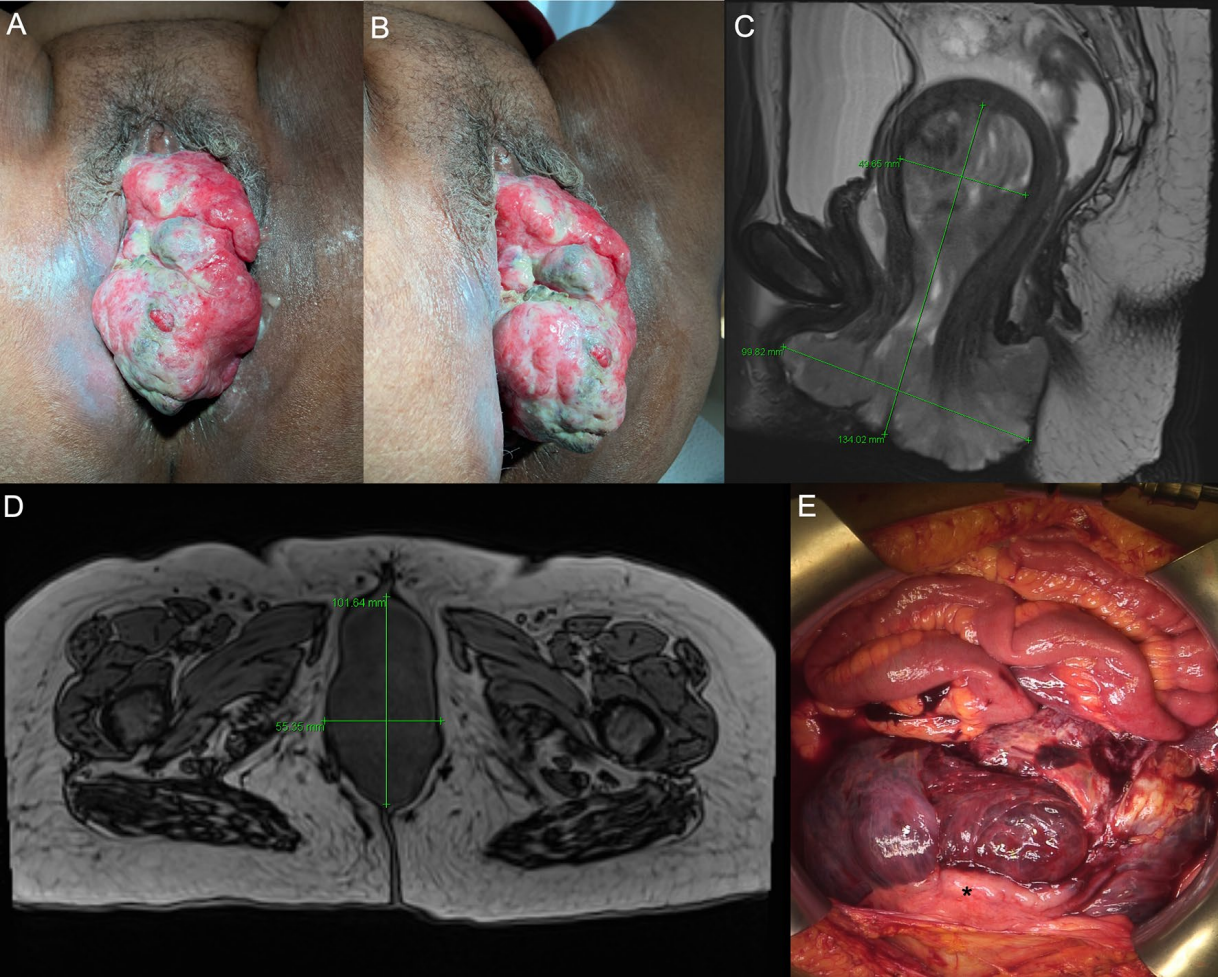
A, B External view of the tumor prolapsing out through the vagina. A View from the front. B View from the right side. C, D MRI scan of the pelvis, scale in mm. C Transverse view, T1 sequence. D Sagittal view, T2 sequence. E Intraoperative situs. 12 o’clock: cranial, 6 o’clock caudal. Lower abdomen filled with a gelatinous tumor. *Uterus
